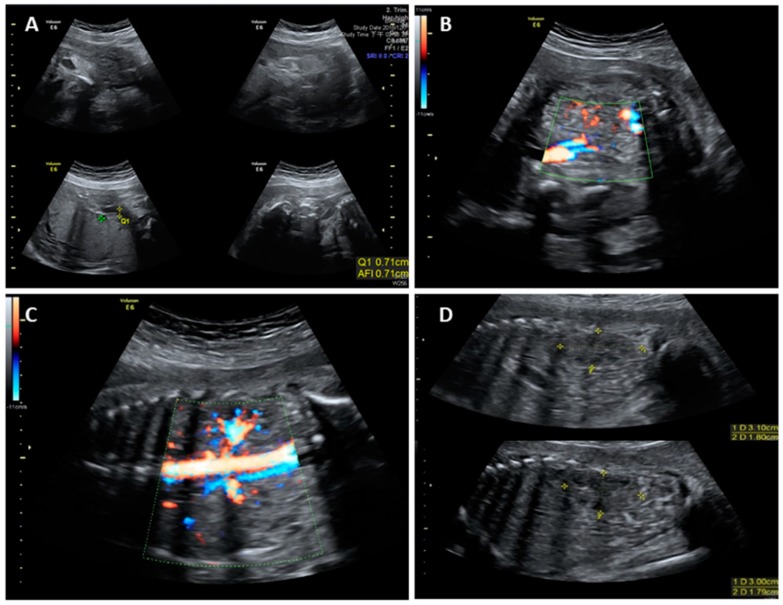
Prenatal ultrasound images of the fetus with RTD at 28^+6^ wGA showing (A) severe oligohydramnios (amniotic fluid index, AFI = 0.71), (B) invisible bladder, (C) visible bilateral renal arteries, and (D) morphologically normal kidneys with a proper size and corticomedullary differentiation (upper: right kidney; lower: left kidney).
